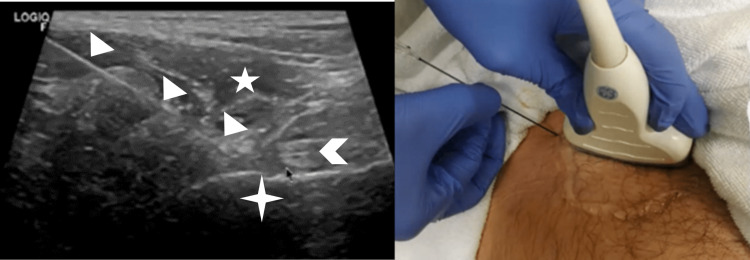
Ultrasound-guided radiofrequency ablation technique On the left is a transverse ultrasound image of the target to perform the radiofrequency ablation of femoral nerves. The patient is in prone position. Arrowhead: psoas tendon, triangular shape: needle, four-pointed star: pubic ramus, five-pointed star: iliacus muscle
